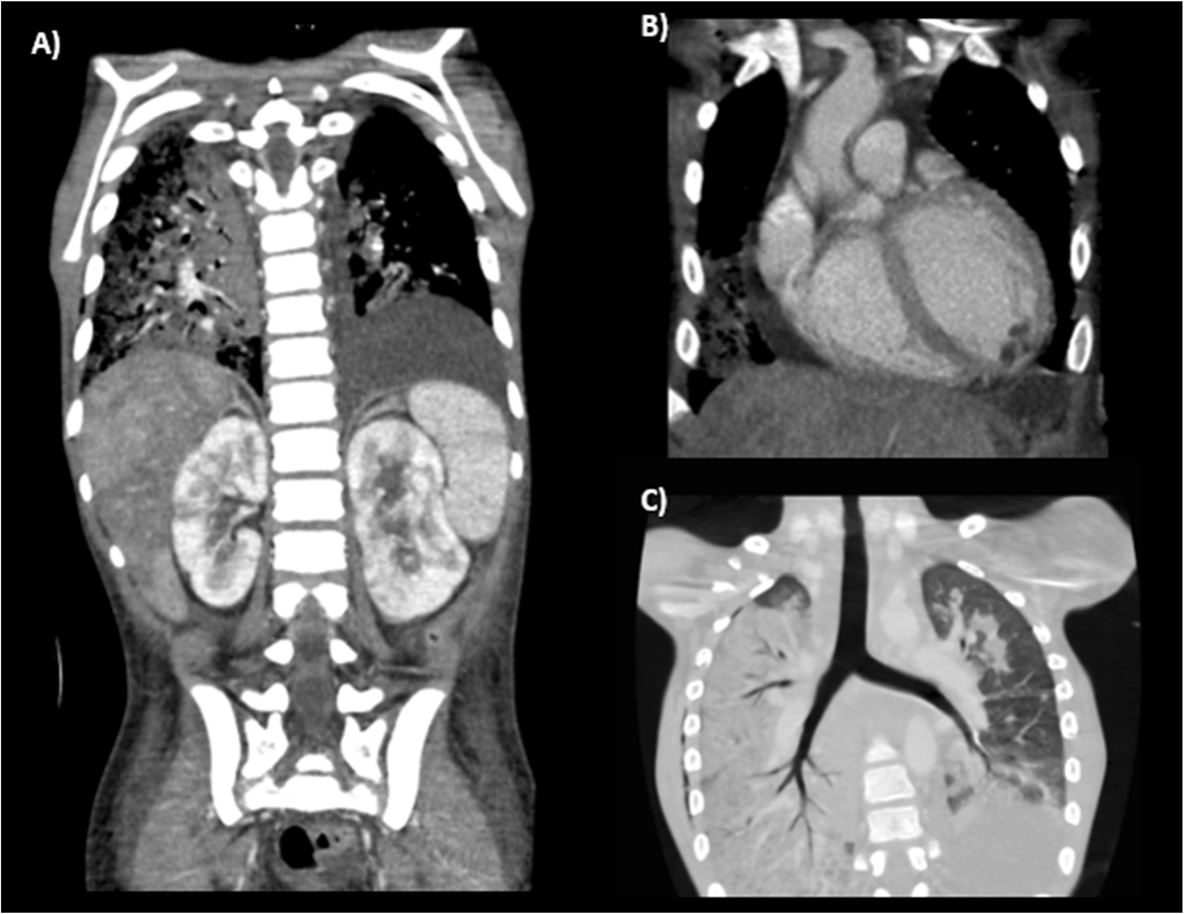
Coronal contrast-enhanced CT of the chest and abdomen. A and B) Soft tissue, and C) Lung algorithm. Images show extensive alveolar infiltrates with hepatisation of both lungs and moderate left pleural effusion. Diffuse cardiomegaly and hypovascular nodules located in septal and left apical ventricular subendocardium. Multiple ill-defined hypodense lesions predominantly involving the periphery of the liver, kidneys, and spleen. Enlarged mediastinal lymph nodes were also present (not shown)
